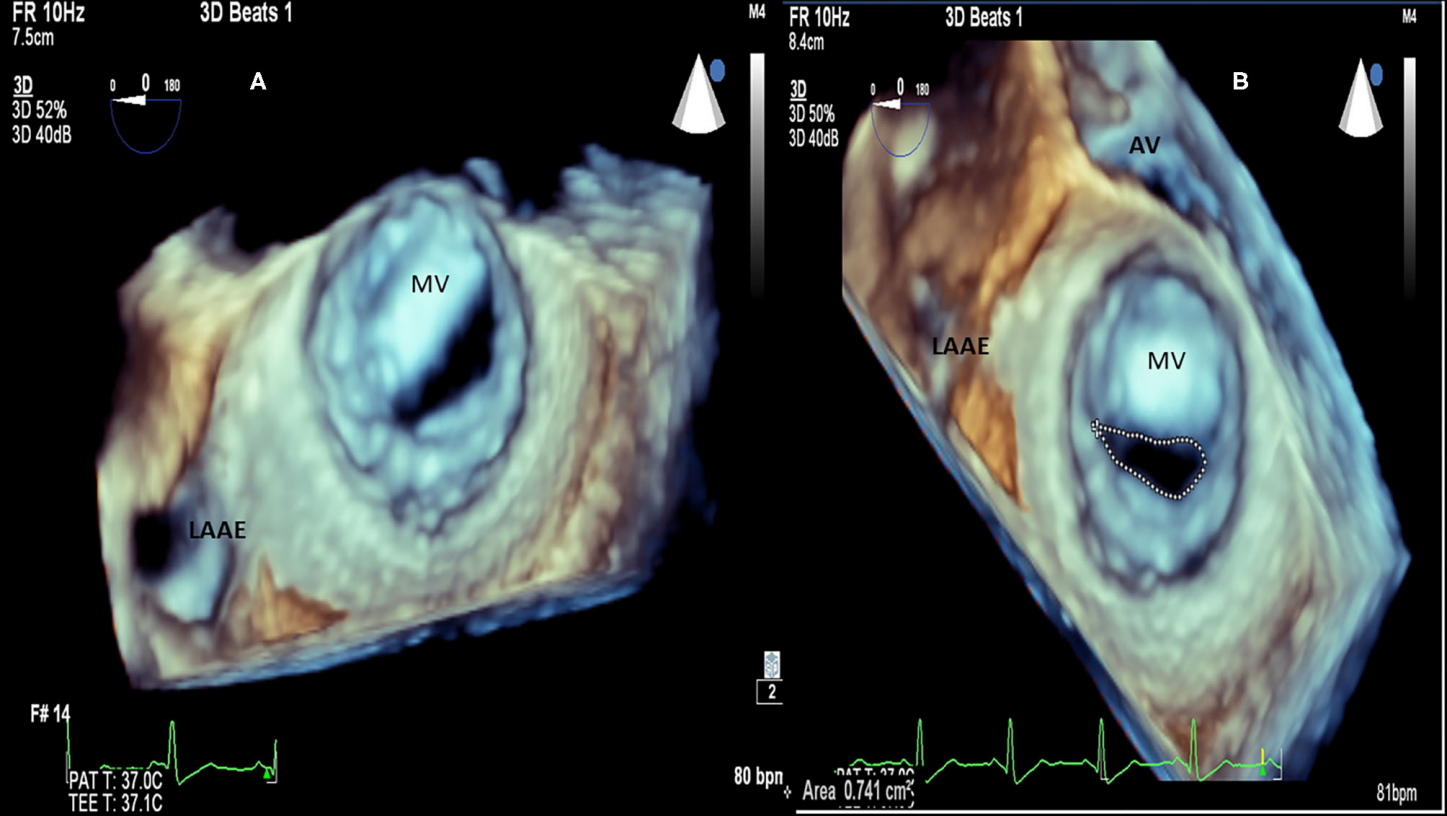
RHD (Mitral valve stenosis). (A) 3D transesophageal echocardiography. Demonstration of patient with severe mitral valve stenosis, “fishmouth” shape of the mitral valve. (B) 3D transesophageal echocardiography, same patient, measurement of the mitral valve area by planimetry: 0.741 cm^2^. LAAE, left atrium appendage; AV, aortic valve; MV, mitral valve.
